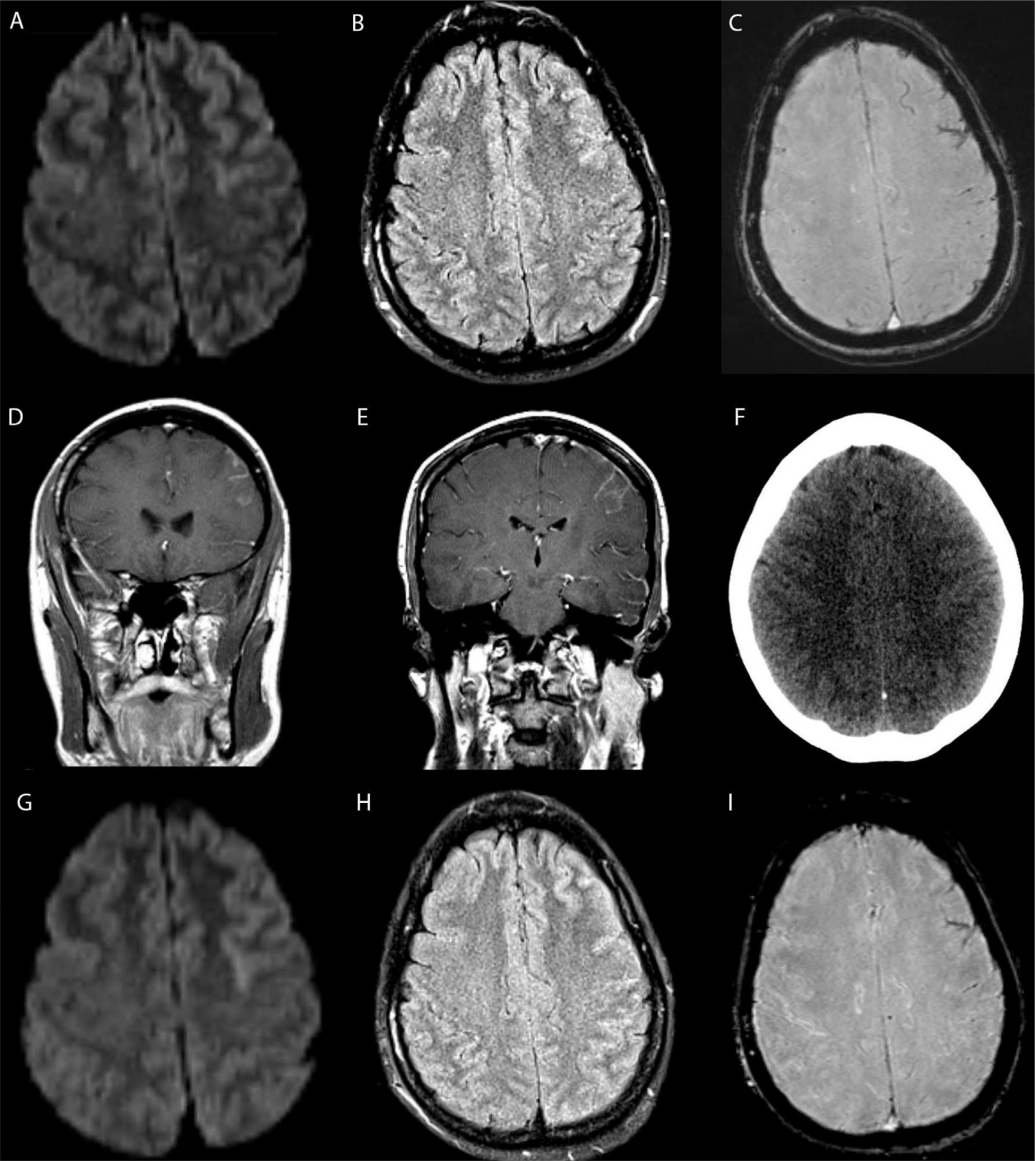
Imaging studies from Case 1. 3.0 Tesla MRI performed 2 hours after symptom onset demonstrates no acute infarction on DWI (A) and subtle FLAIR non-suppression (B), susceptibility artifact (C), and contrast enhancement (D, E) in the left cerebral hemispheric sulci. Non-contrast CT (F) performed 13 hours after symptom onset demonstrates subtle edema and sulcal effacement in the left cerebral hemisphere. 1.5 Tesla MRI performed 14 hours after symptom onset again demonstrates no acute infarction on DWI (G) but FLAIR non-suppression (H) and susceptibility artifact (I) in a left fontal sulcus, compatible with extravasated contrast or trace subarachnoid blood.
